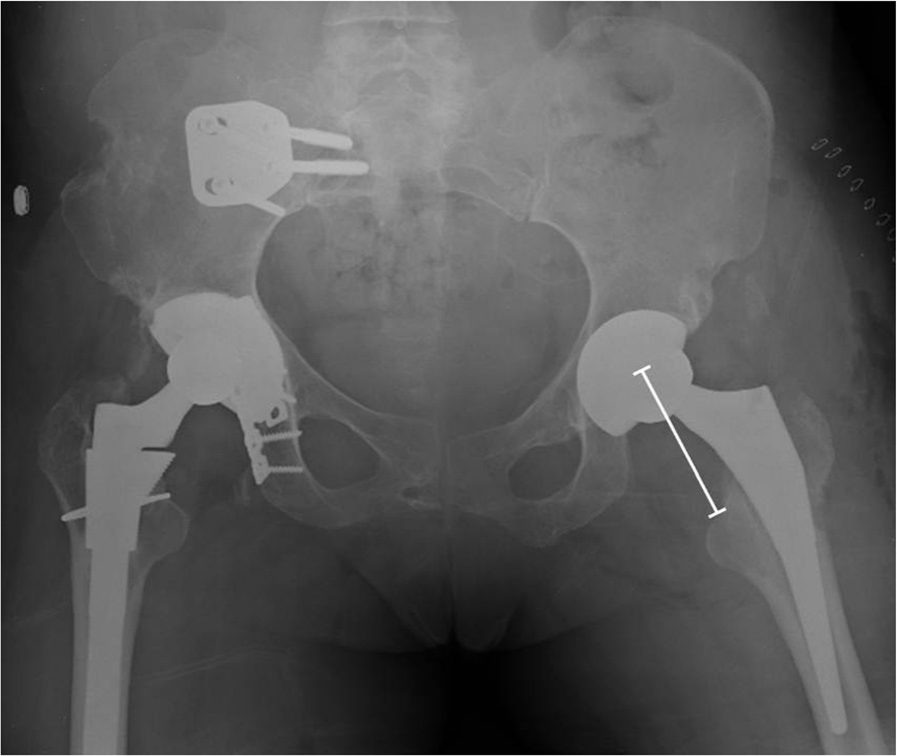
Anteroposterior pelvic radiograph after the 2nd surgical revision of the left hip with a total hip arthroplasty. Note the distance between femoral head center of rotation to the lesser trochanter.
